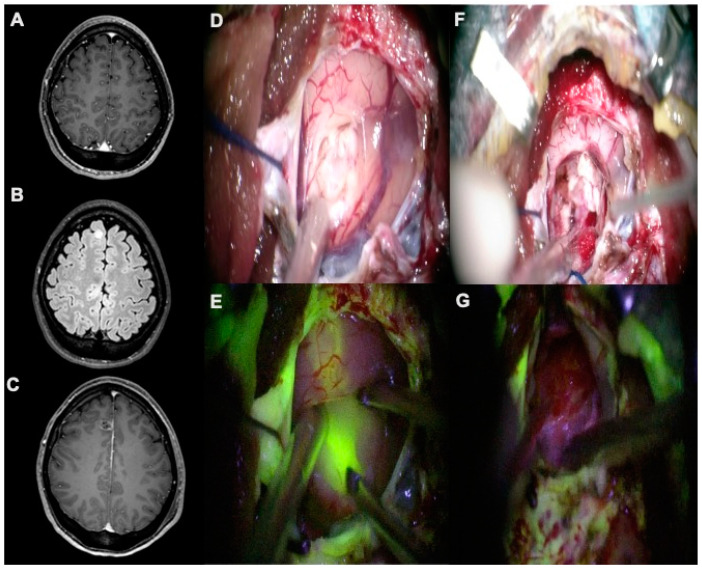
(A,B) Preoperative, postcontrast axial T1-weighted and axial FLAIR-weighted scans showing a right frontomesial ganglioglioma, completely removed as detectable by postoperative, postcontrast axial T1-weighted scan (C). (D,E) During surgical resection, YELLOW 560 filter highlighted pathologic tissue allowing the identification of a clear plane of dissection by using the fluorescent visualization. (F,G) At the end of resection, the cavity did not show any sign of a residual tumor, as confirmed by fluorescent visualization with YELLOW 560 filter.
